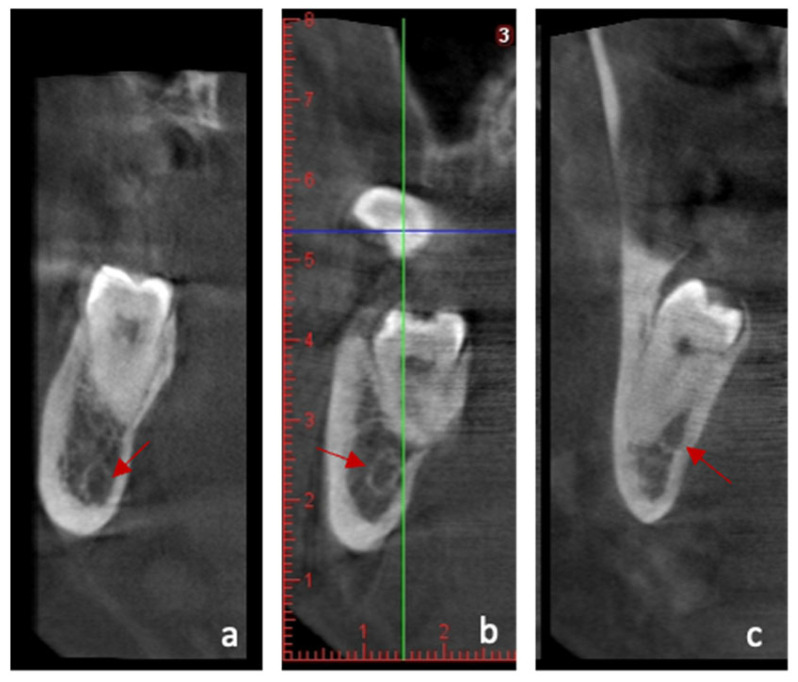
Example of CBCT images that were used to assess the three-dimensional position of M3M and establish possible predictors of surgical risk. For this purpose, three distinct analyses will be performed: position of IAC in relation to the roots of M3M: (a) apical; (b) buccal; (c) lingual. The arrow indicates the position of IAC.
